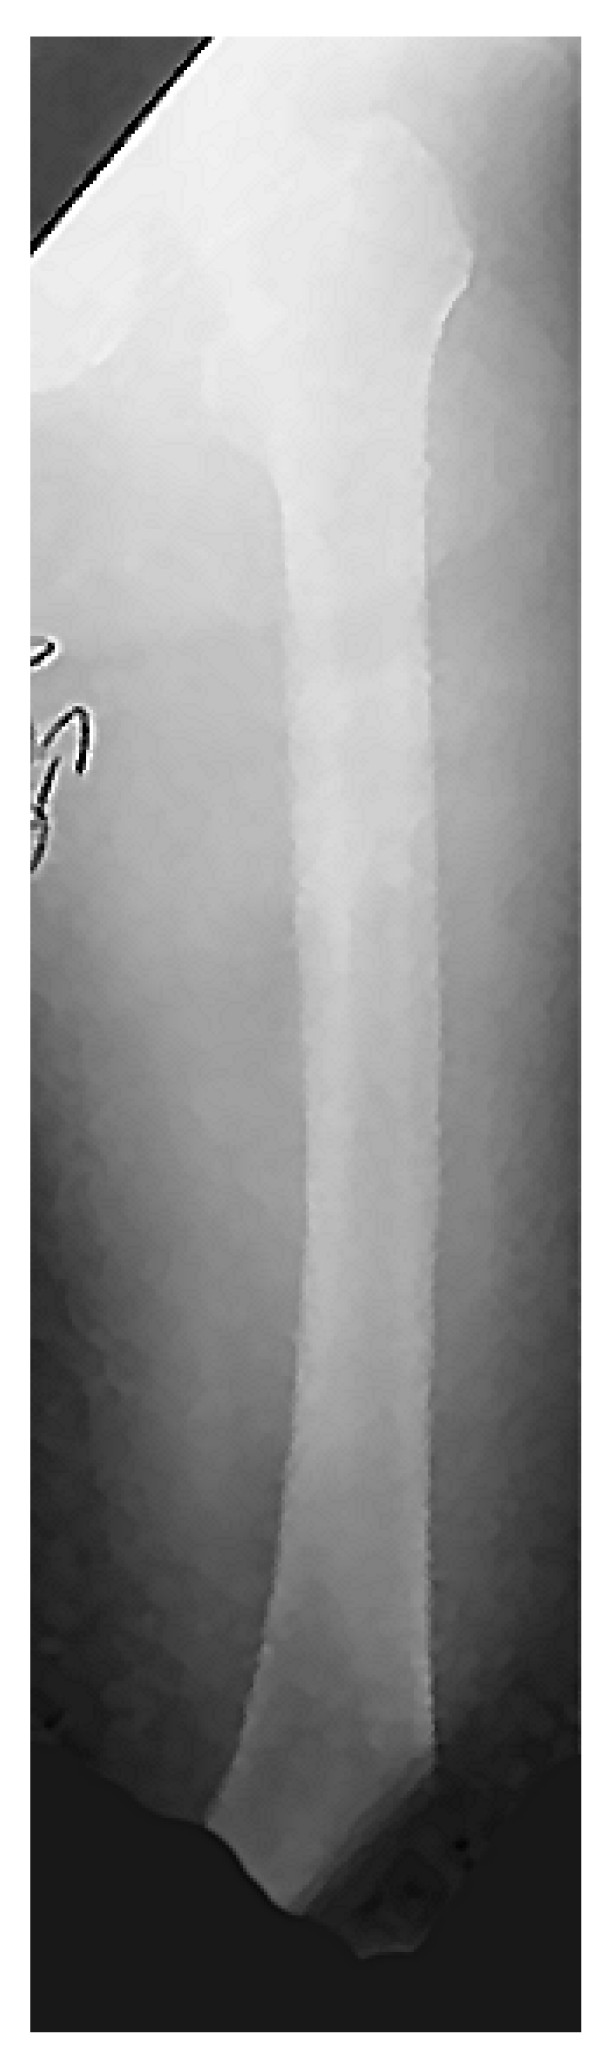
Antero-posterior femur x-ray has not revealed any fracture.
